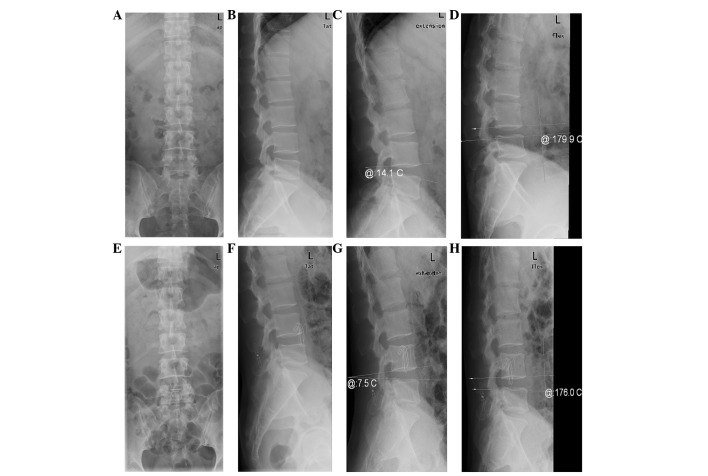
X-rays of (A) a pre-operative neutral position and (B) a pre-operative lateral position to measure the distance between spinous processes, the width and height of the intervertebral foramen, the height of the intervertebral anterior and posterior margins, as well as the lumbar segmental lordosis angle. (C) Pre-operative hyperextension and (D) hyperflexion positions showed that the segments mobilities were >11° and enabled measurements and comparisons of the corresponding distances. (E) In-space position was observed in the post-operative neutral position whether the lateral wings had been completely opened or crimped. (F) Post-operative lateral position showed increases in the distance between spinous processes, the width and height of the intervertebral foramen and the height of the intervertebral posterior margin, as well as decreases in the height of the intervertebral anterior margin and the lumbar segmental lordosis angle. (G) Pre-operative hyperextension and (H) hyperflexion positions showed that the mobilities of the abnormal segments were significantly decreased.
